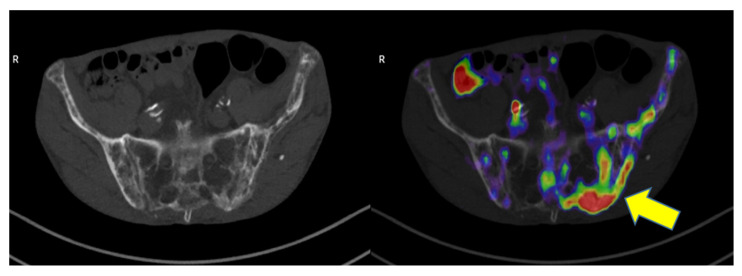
An example of Paget disease in CT (on the left) with increased [^18^F]FDG uptake (yellow arrow) at PET/CT (on the right).
